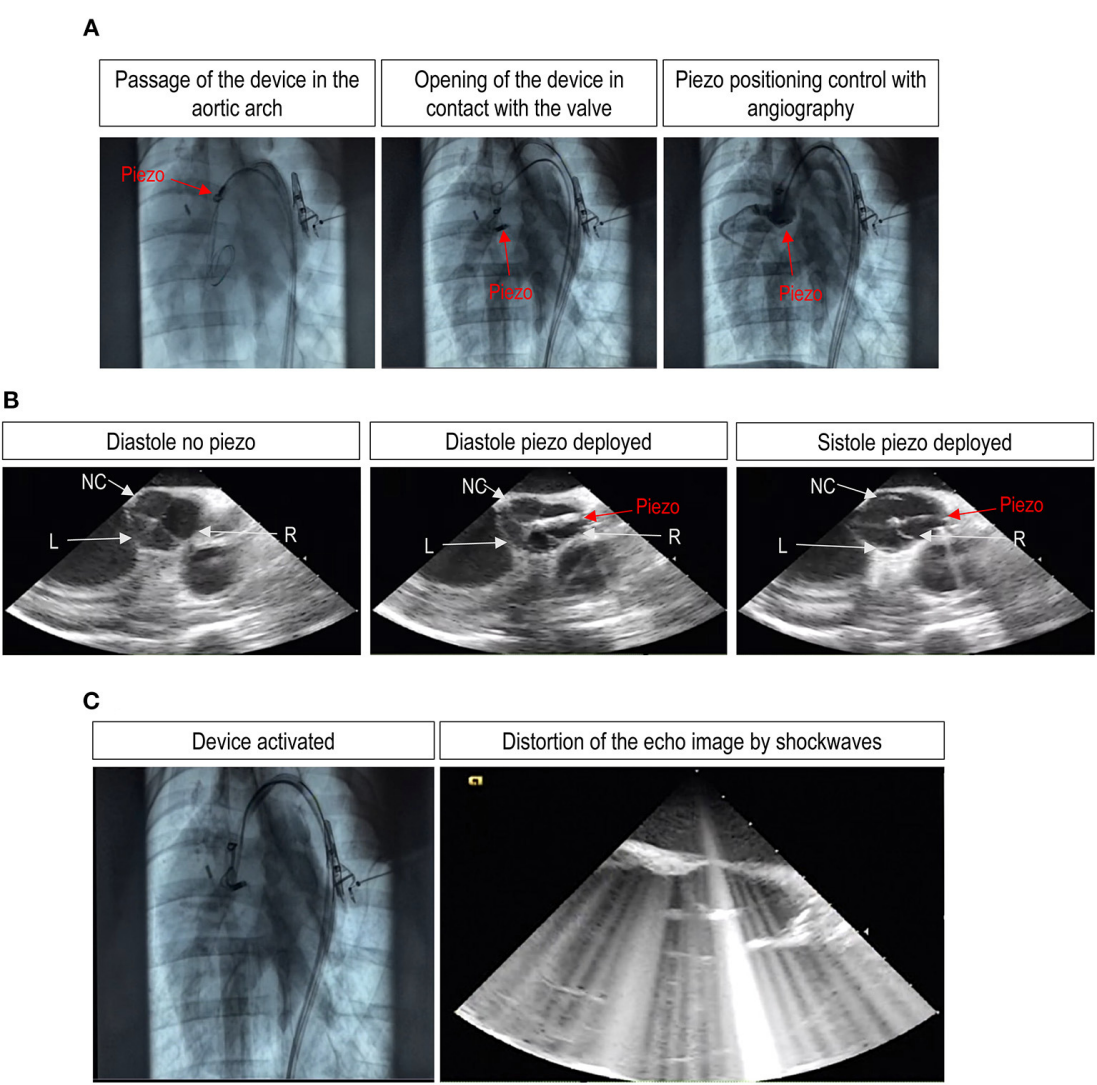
Angiographic and echocardiographic assessment of successful TDD deployment and leaflet treatment with a trans-femoral access *in vivo*. (A) Shows the deployment of the device through the femoral artery from an initial position at the level of the ascending aorta and down to the positioning of the piezo in the Valsalva sinus of the right aortic valve leaflet. (B) Shows three images of an echocardiographic sequence showing the motion of the valve leaflets in the presence of the piezo deployed at the level of the right leaflet. The pictures of the sequence show, respectively, the correct closure of the three leaflets before piezo deployment (left picture), the closure of the valve after piezo deployment (center picture), and the opening of the left (L) and the non-coronary (NC) leaflets or the blockade of the right (R) leaflet by the deployed piezo at systole (right picture). The complete sequence is provided as Supplementary Video 1. (C) Shows, finally, static images of the angiography and the echocardiographic sequences during activation of the piezo. It is evident on the right the interference of shockwaves with the ultrasounds, making it impossible to distinguish the structure of the valve.
